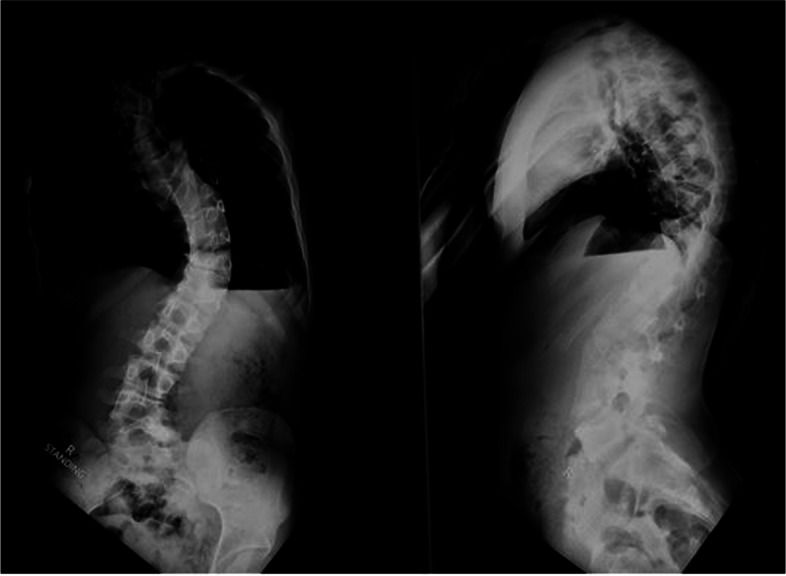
Pre-operative standing, full length x-ray for scoliotic curve assessment. Cobb’s angle of 70 degrees was seen due to congenital scoliosis with D3, D7, D9 and D12 hemivertebra and fusion of right sided posterior element of D3 and D4 vertebra
